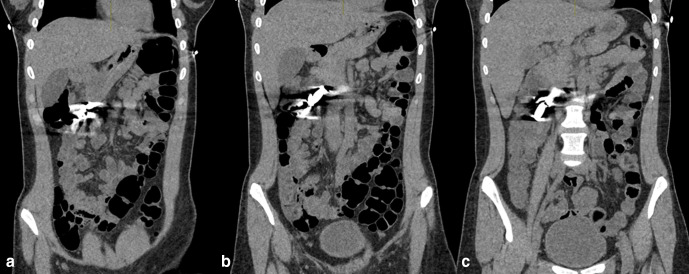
a,b,c: Sequential non-contrast coronal CT images, demonstrating the aggregation of button magnets. No metal reduction artefact applied.
